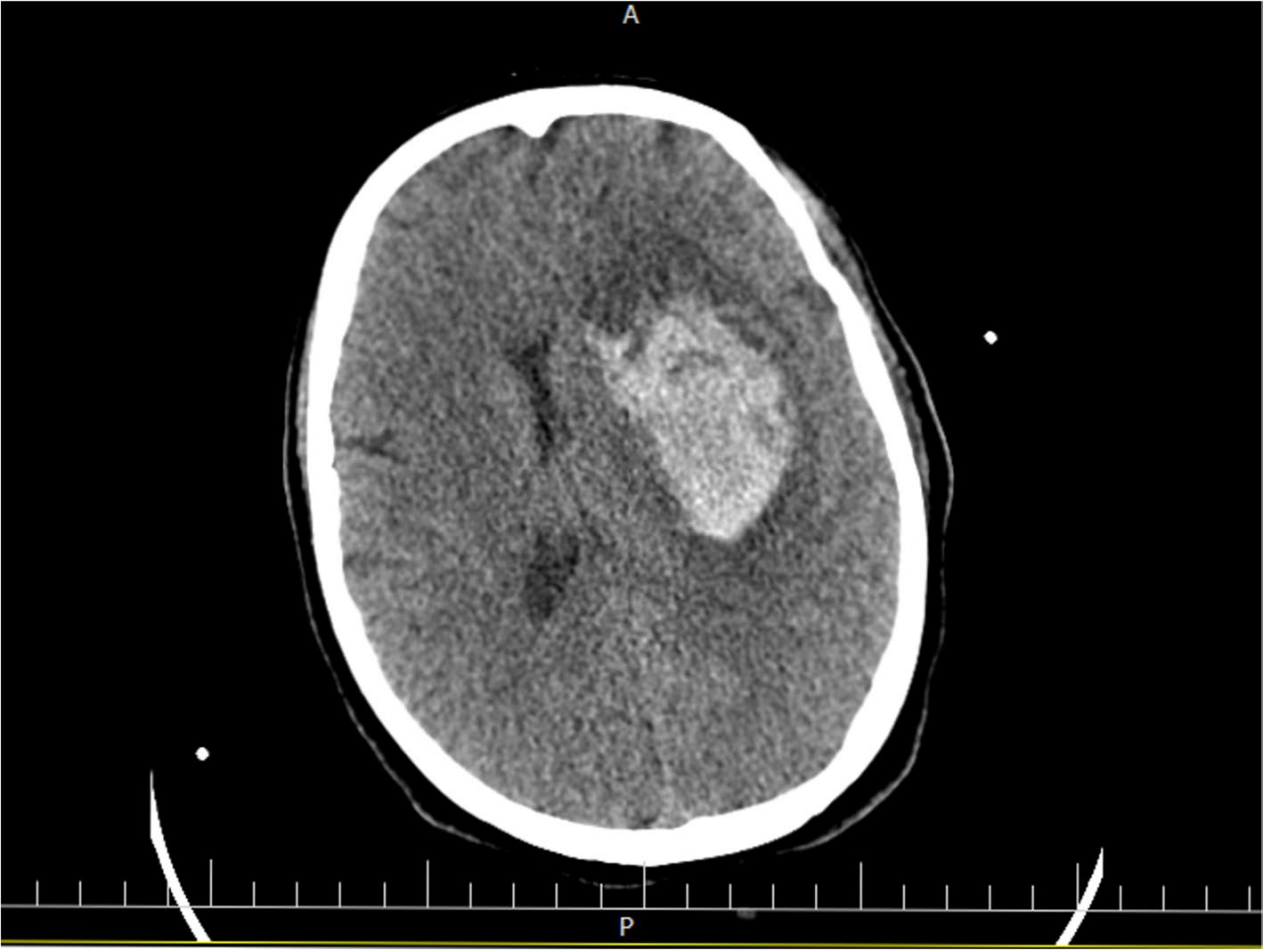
Axial view of brain CT scan showed a 30*56 mm hyperdense mass-like lesion in the left fronto-parietal area, extensive hemorrhage in left basal ganglia and internal capsule, with midline shift and mass effect
